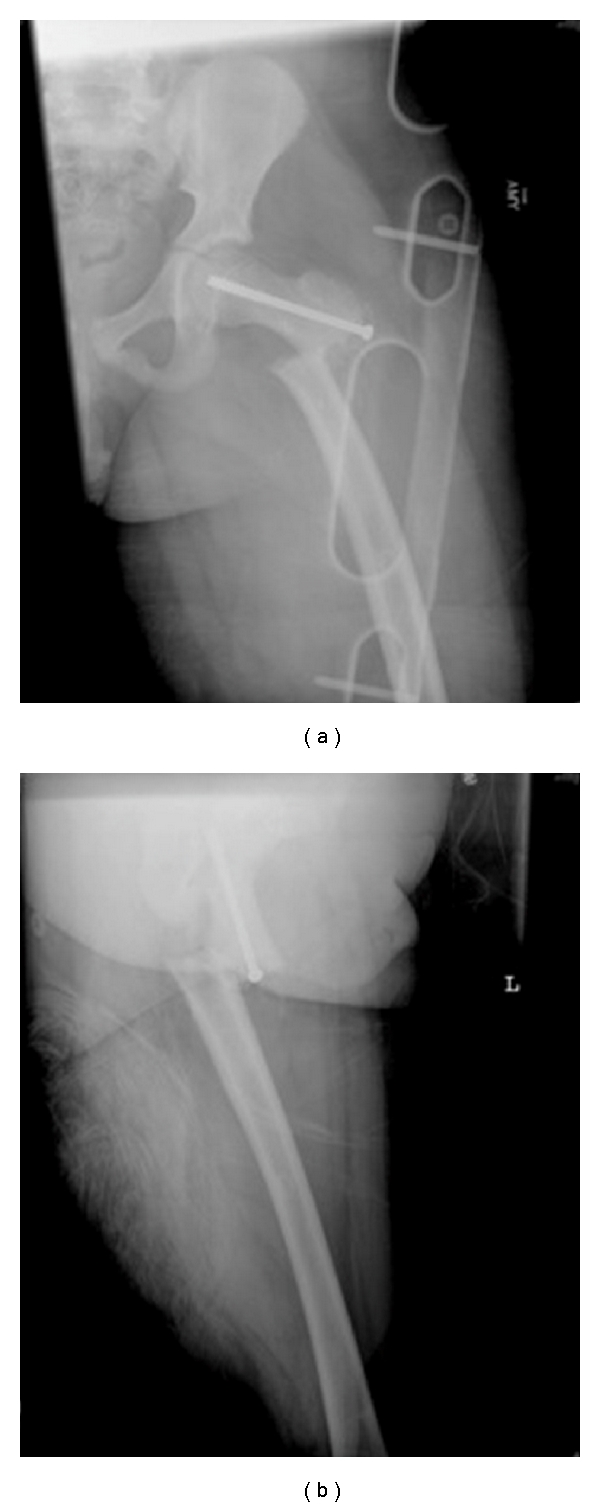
AP (a) and lateral (b) radiographic views of the left hip and thigh after the patient fell off his bicycle.
